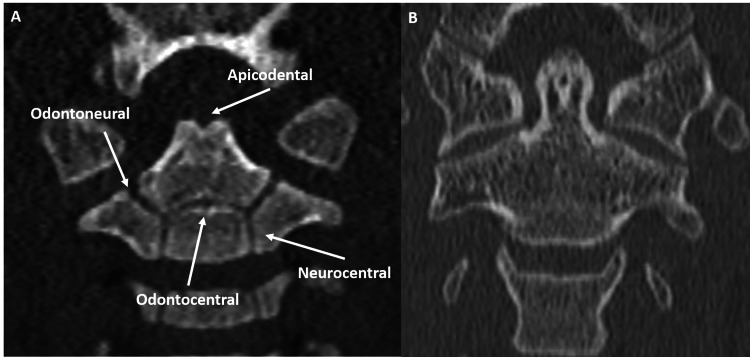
Coronal CT image demonstrates a normal developing C2 vertebra in a two-year-old (A). The os odontoideum has not yet ossified, but the apicodental synchondrosis is appreciable. The odontocentral is the other midline synchondrosis. The remaining odontoneural and neurocentral synchondroses are symmetric. Coronal CT image depicts adult C2 vertebra (B) on coronal axis for comparison. Note apical ossification and complete vertebral fusion. CT - computed tomography
